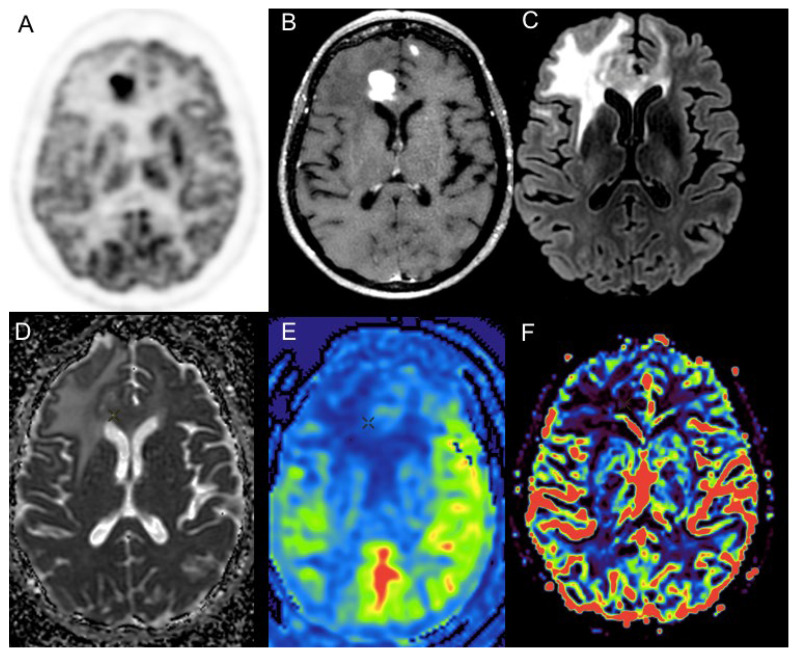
Primary central nervous system lymphoma in a 72-year-old female patient with typical PET and MRI features: high and homogeneous ^18^F-FDG PET uptake (SUVmax = 27, T/N = 2.1) (A); “snowball” pattern with intense and homogeneous enhancement after gadolinium enhancement (B); extensive hypersignal on T2-FLAIR weighted images, respecting perilesional edema and respecting the cortical ribbon (C). Parametric ADC map show only moderate diffusion restriction (D), and no hyperperfusion is detectable either on arterial spin labelling (E), or dynamic susceptibility contrast (F, rCBV parametric map) perfusion sequences.
